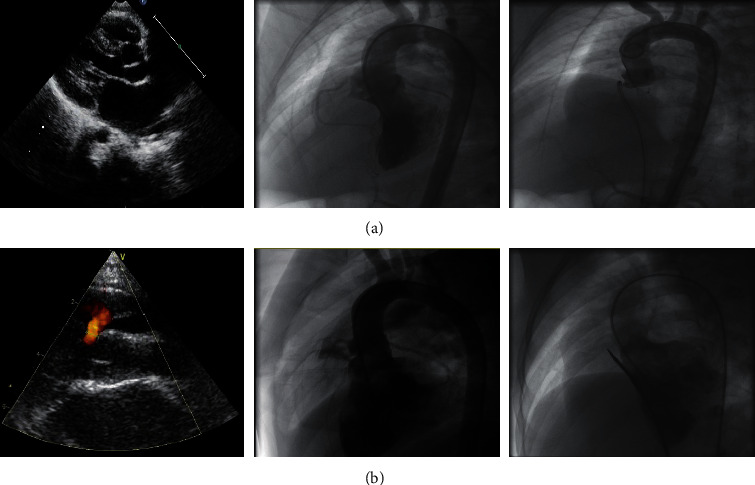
(a) Ventricular septal defect with severe aortic valve prolapse. The right coronary cusp prolapses into the right ventricular outflow tract (left, center). A 5/4 mm Amplatzer duct occluder II was successfully implanted without aortic regurgitation as shown by the angiography (right). (b) Intracristal ventricular septal defect. Transthoracic echocardiography (left) shows the defect is close to aortic valve. Left ventricular angiography at 70° left anterior oblique and 20° cranial projection was performed to visualize the defect (center). A 5/4 mm Amplatzer duct occluder II completely closed the defect completely without residual shunt or interfering with the aortic valve (right).
